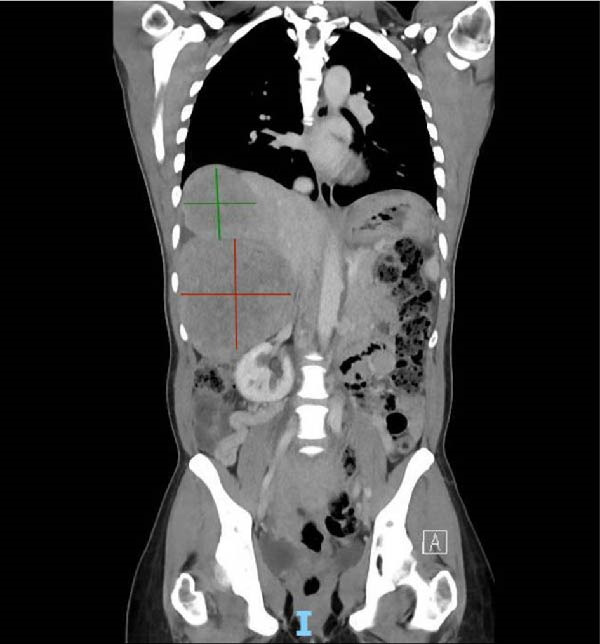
CT abdomen and pelvis coronal view showing superior hepatic mass (marked in green) and inferior hepatic mass (marked in red).
